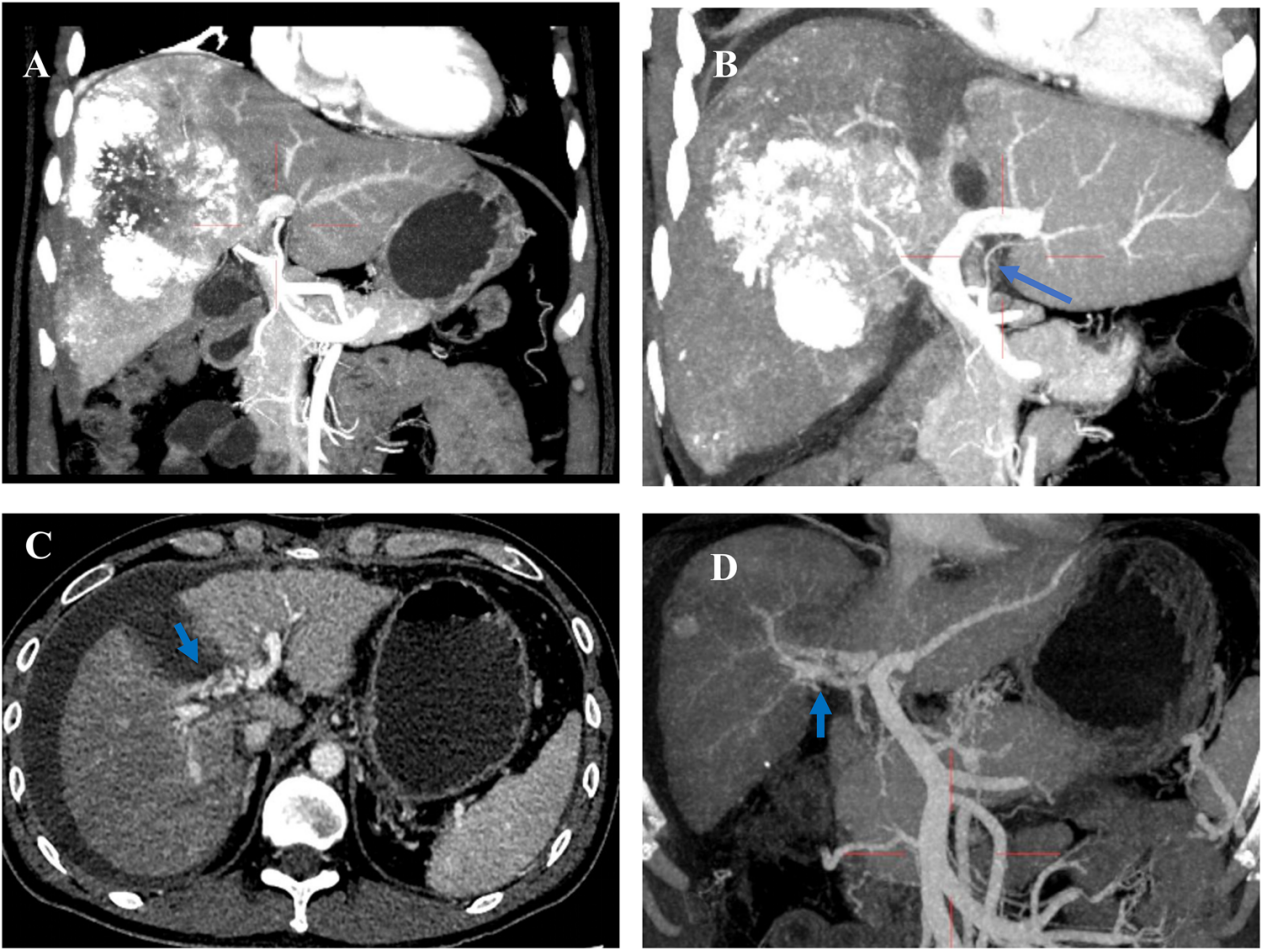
Inadequate liver regeneration due to hemodynamic changes. A A 65-year-old male patient, the maximum intensity projection of the preoperative CT image in the arterial phase clearly shows the hepatic artery; this patient underwent TACE treatment before. B The same patient in A; the maximum intensity projection of the arterial phase CT image 7 days after ALPPS stage 1 shows a smaller left hepatic artery branch (arrow) due to the “arterial steal ” by the huge HCC lesion with inadequate liver regeneration of the left lobe. C A 36-year-old male patient, the axial CT in portal phase in 7 days after ALPPS stage 1 shows recanalization of the right portal vein due to the arteriovenous fistula (arrow) between the right hepatic artery with right portal vein. D The same patient in C; the maximum intensity projection of the portal phase CT image 7 days after ALPPS stage 1 identifies the recanalization(arrow) of the right portal vein
